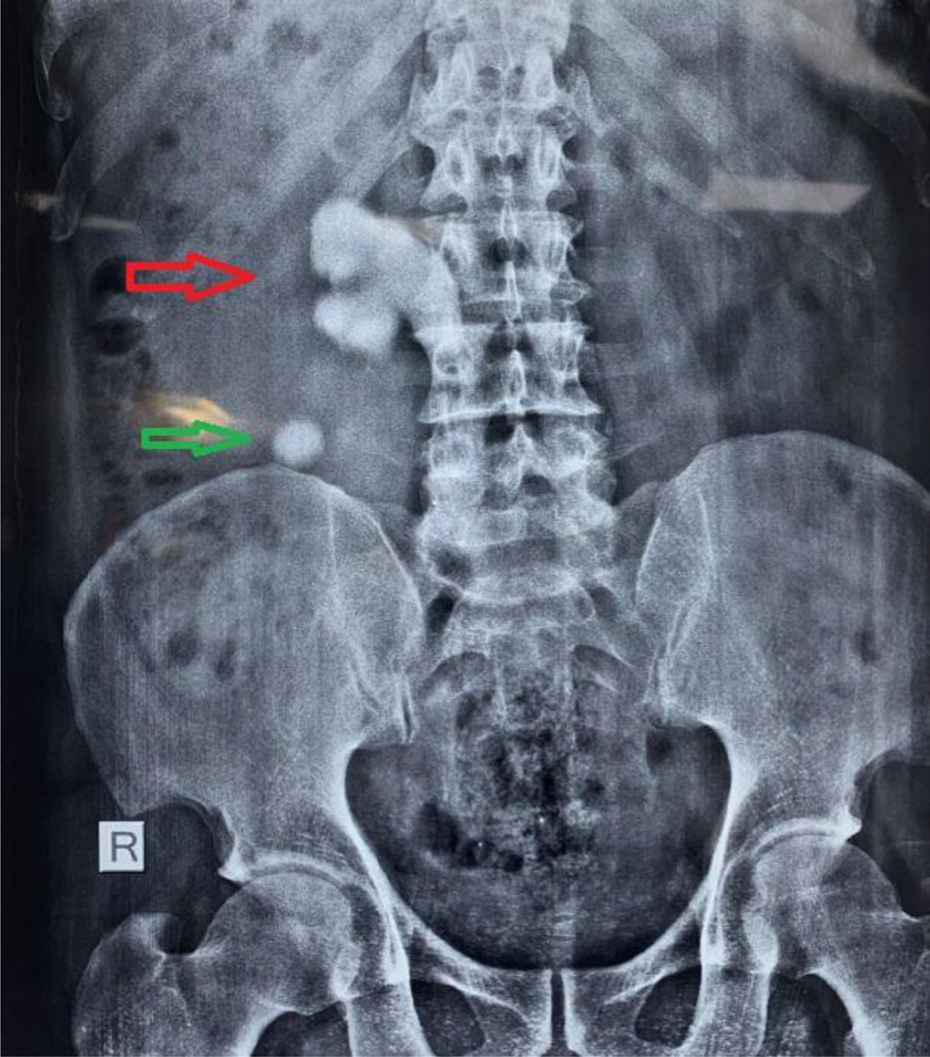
Plain x-ray KUB showing a huge renal pelvic stone extending to the upper ureter and another small stone in the lower calyx, depicted by red and green arrows, respectively.
